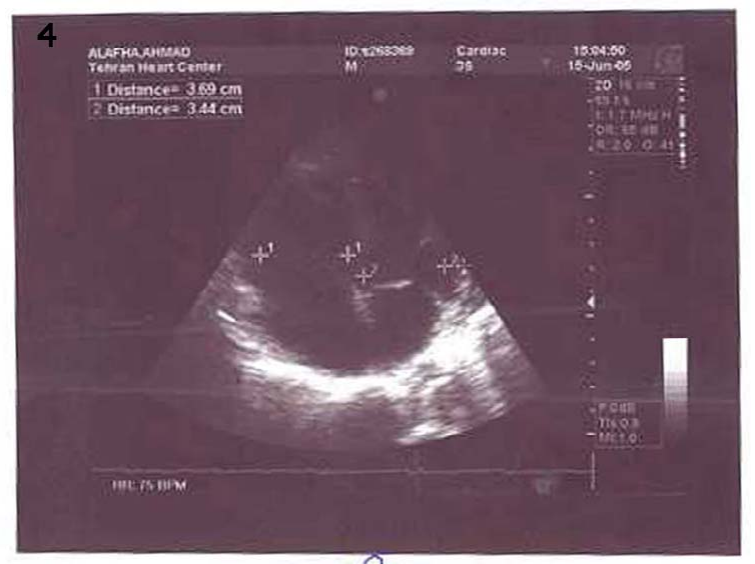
echocardiography shows disappearing of clots after treatment.
